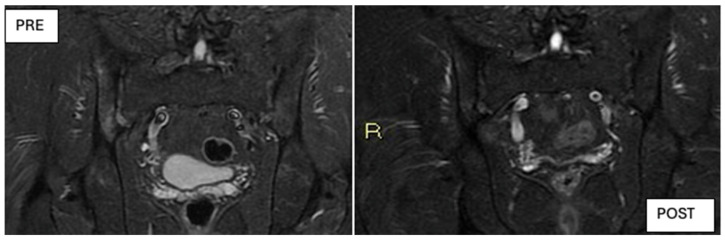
MRI images at baseline (t_0_, left) and after 6 months of treatment with Guselkumab (t_6_, right) showing a clear reduction in bone marrow edema and inflammatory lesions.
